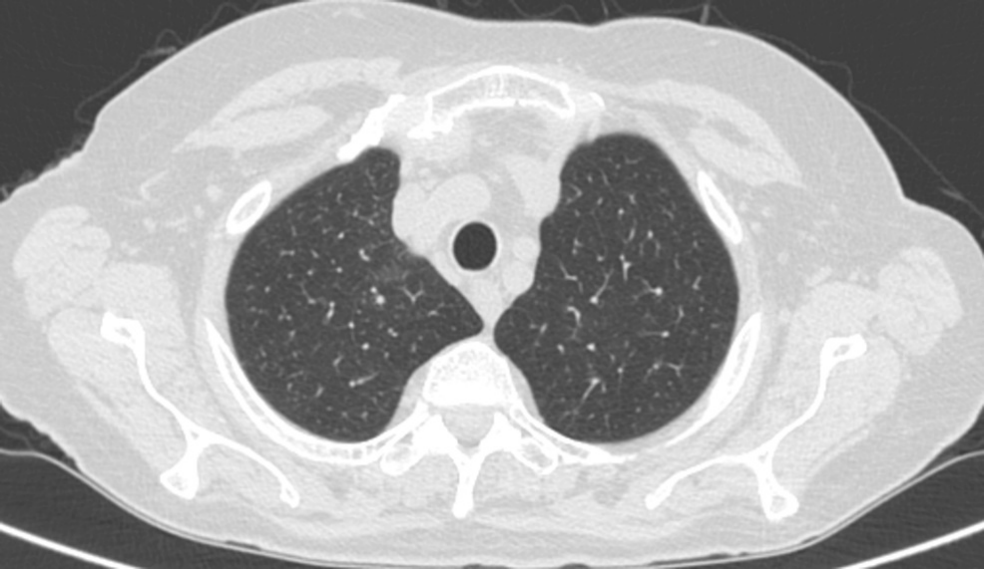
High-resolution chest CT showing diffuse centrilobular micronodules.
